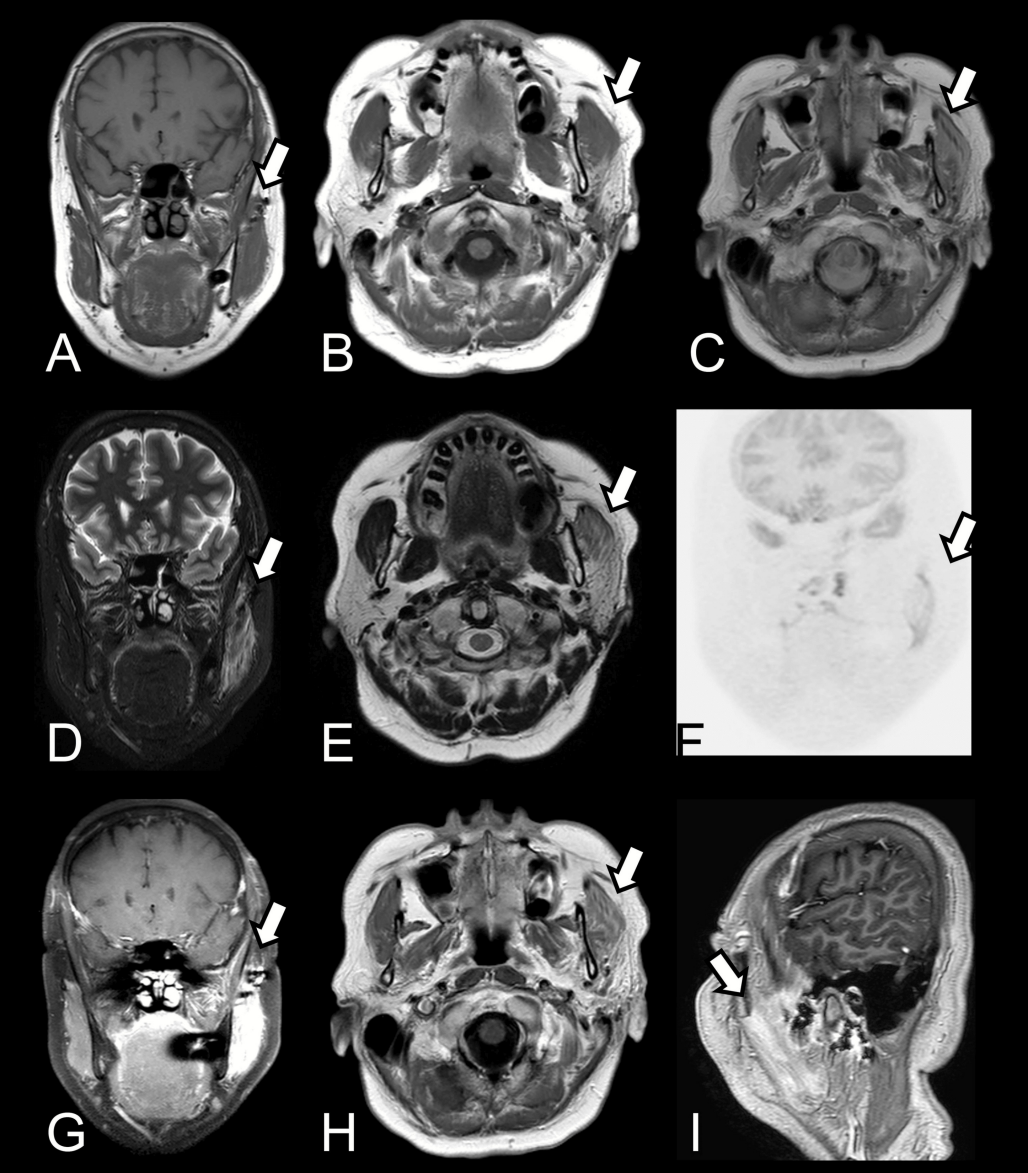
MRI at the three-month postoperative follow-up. (A and B) T1-weighted, (C) proton density, (D) STIR, (E) T2-weighted, (F) diffusion-weighted, and (G, H, and I) post-contrast images indicate status post operation without definite residual tumor in between the left masseter muscle and left parotid gland. Hyperintense T2 signal change in the left masseter muscle, probably due to postoperative inflammatory process. MRI: magnetic resonance imaging; STIR: short tau inversion recovery
